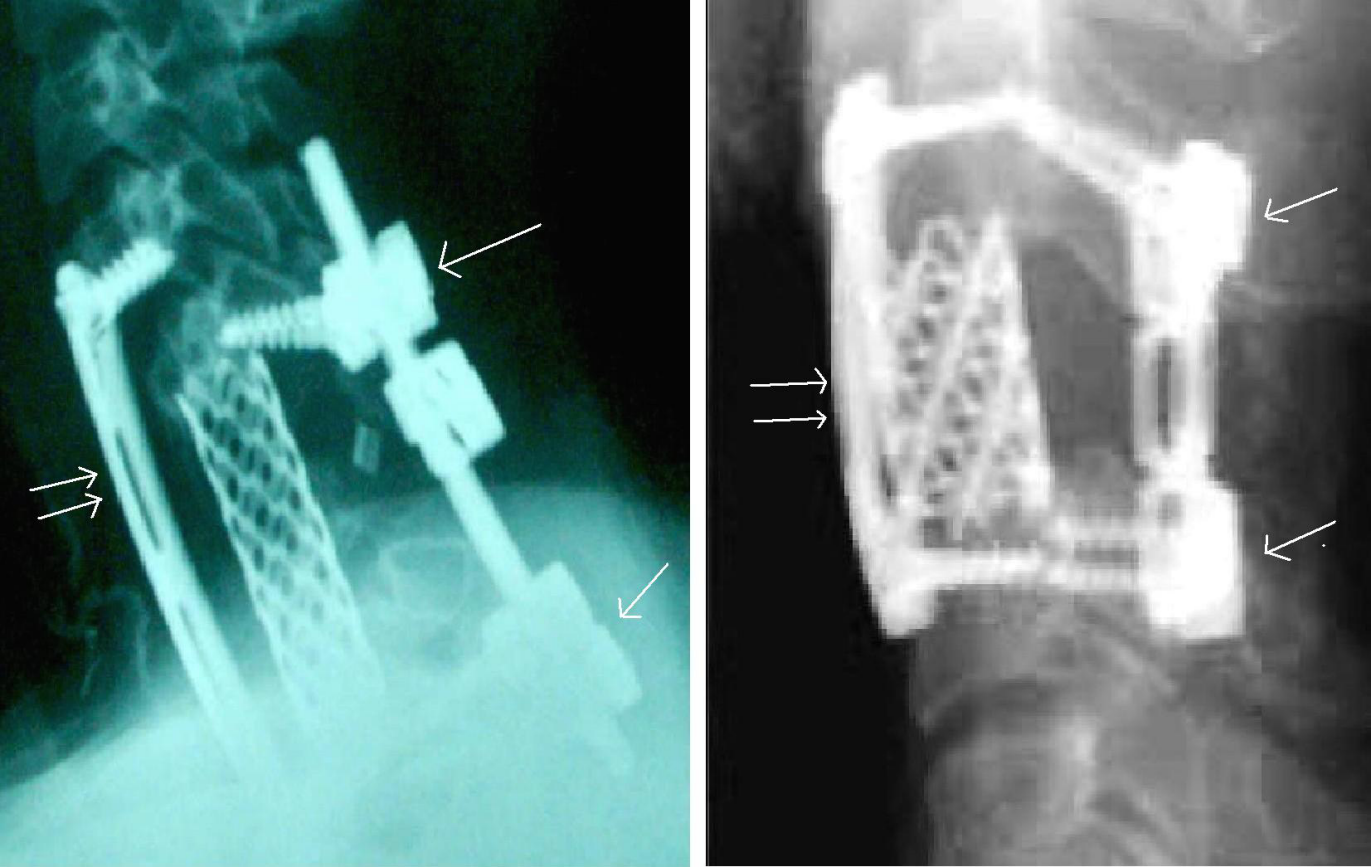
Post operative lateral X-rays. Anterior and posterior spinal approaches with corpectomy and total resection of the spinal tumor from front and back in a staged operation with lateral mass fixation.
